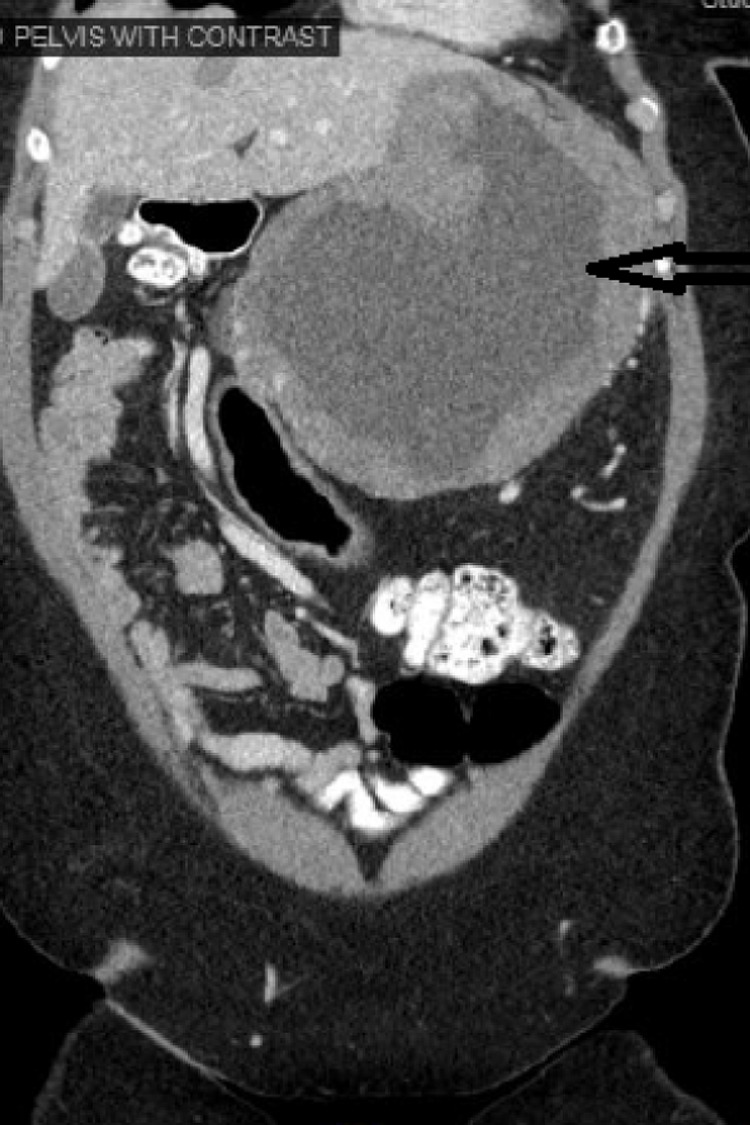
Computed Tomography of the abdomen and pelvis with coronal view Black arrow pointing to the mass
